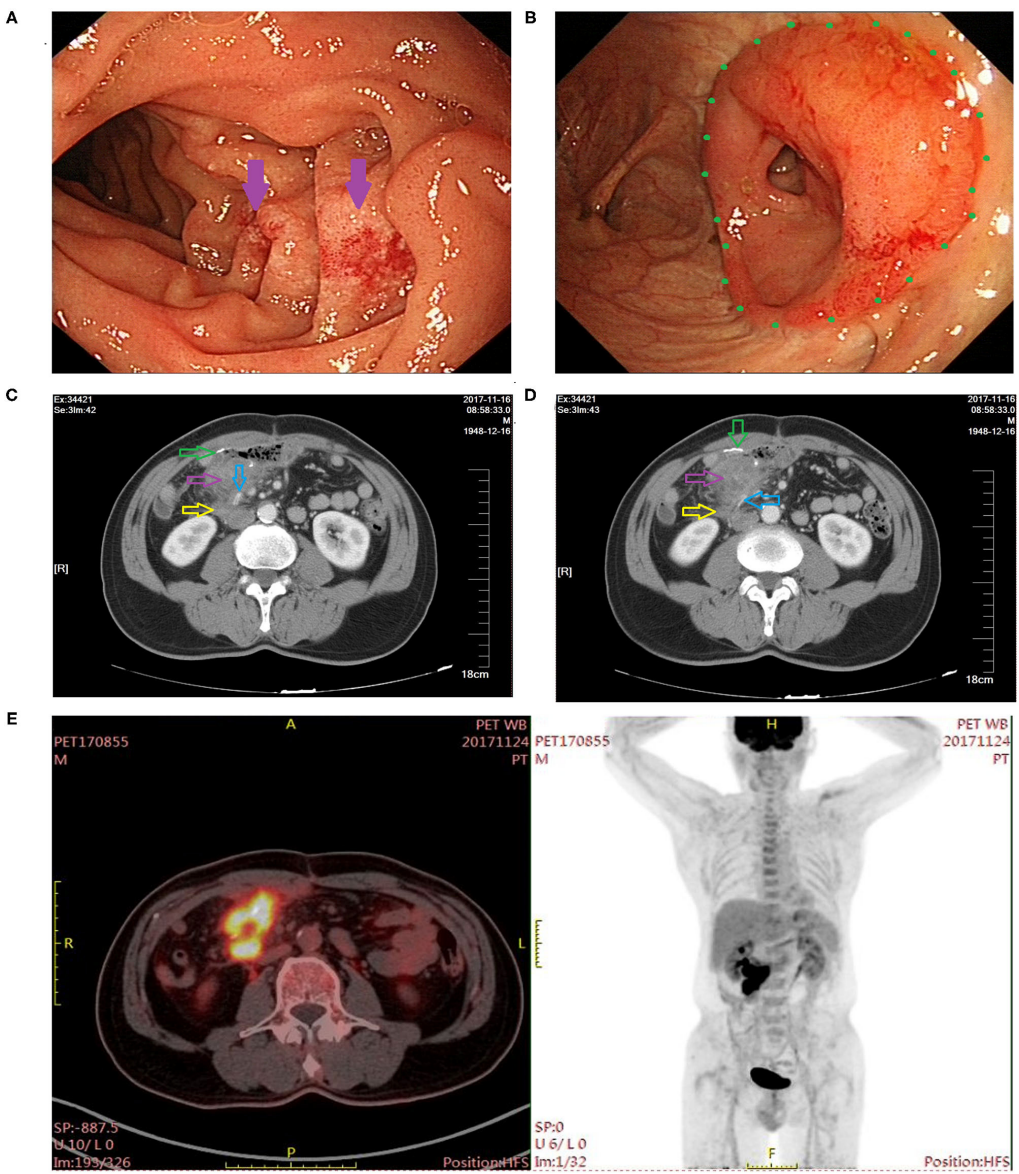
Postoperative imaging findings at 8 months after right hemicolectomy. (A) Gastroscopy revealed inflammatory changes in the descending part of the duodenum (indicated by the purple arrow). (B) Colonoscopy showed inflammatory changes at the ileocolic anastomosis site (indicated by the green dot). (C,D) CT examination showed exudation around the ileocolic anastomosis (the green arrow indicates the ileocolic anastomosis; the purple arrow indicates inflammatory exudate; the yellow arrow indicates the duodenum; and the blue arrow indicates the vascular clip). (E) PET-CT showed high concentration of radioactivity around the anastomosis.
